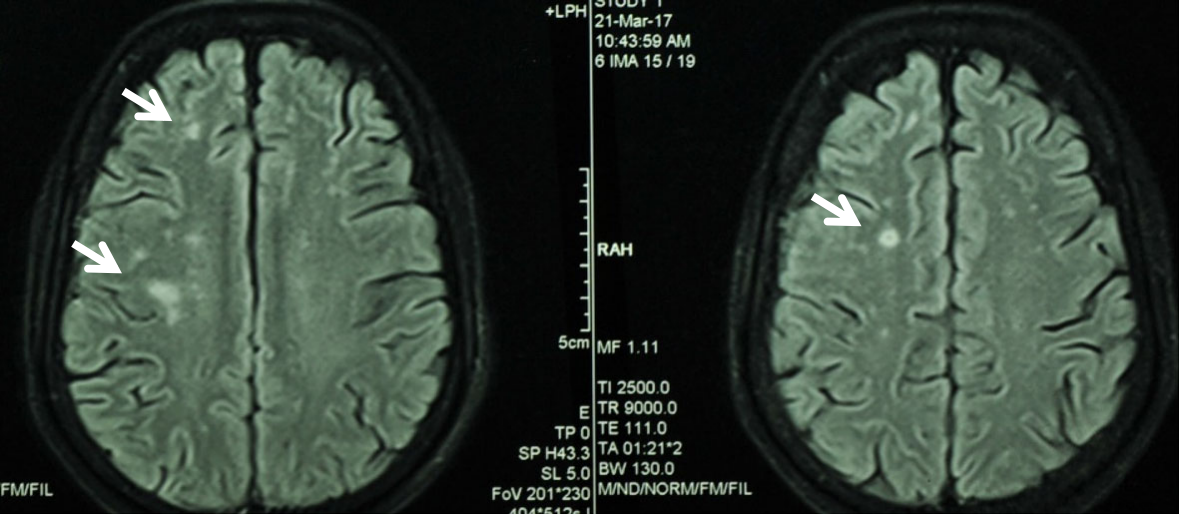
MRI brain showing multiple cortical infarcts (white arrows)
